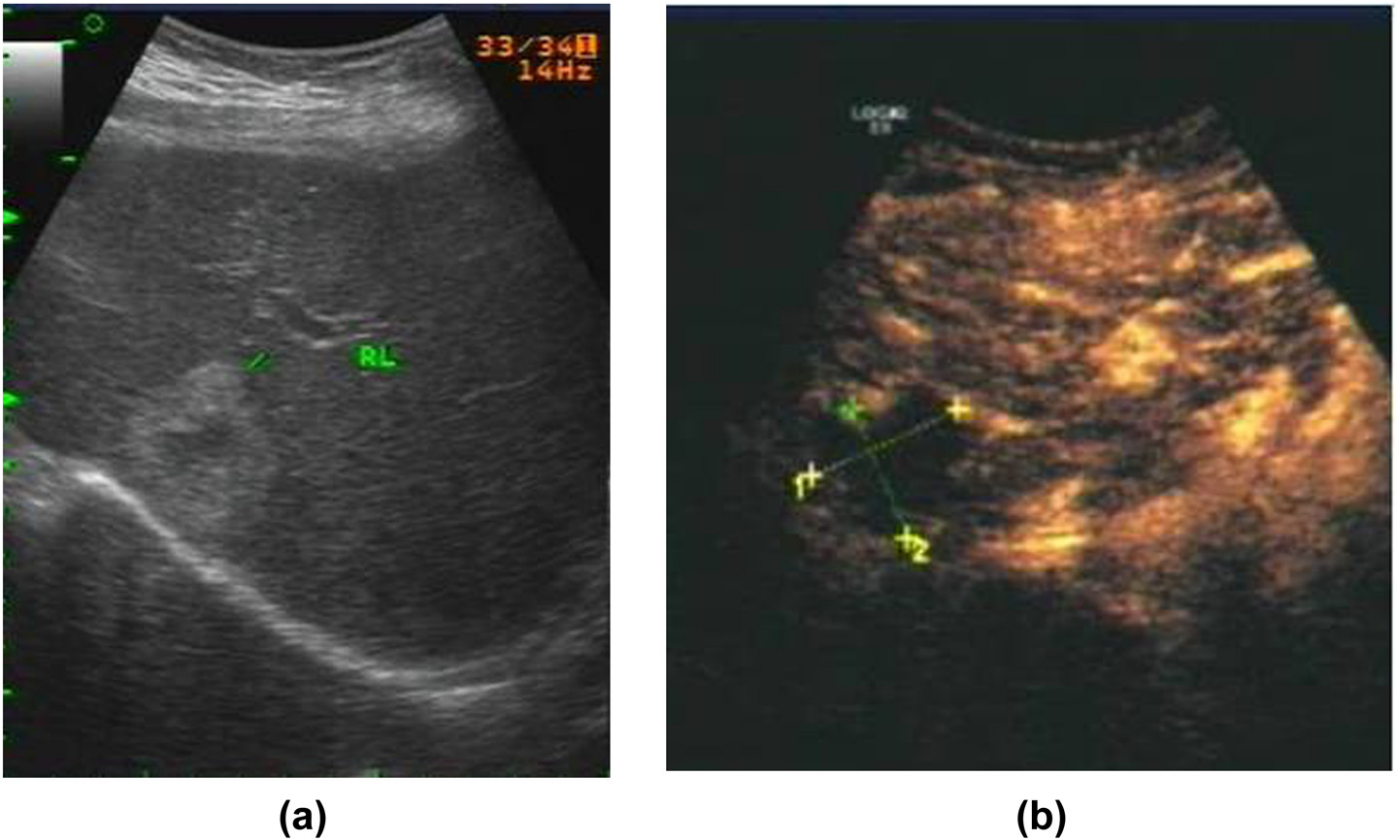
(a) Two-dimensional ultrasound imaging shows an irregular hyperechoic lesion in the right lobe of the liver with liquefaction in the center. (b) Contrast-enhanced ultrasound shows no contrast enhancement in any of the three phases.
